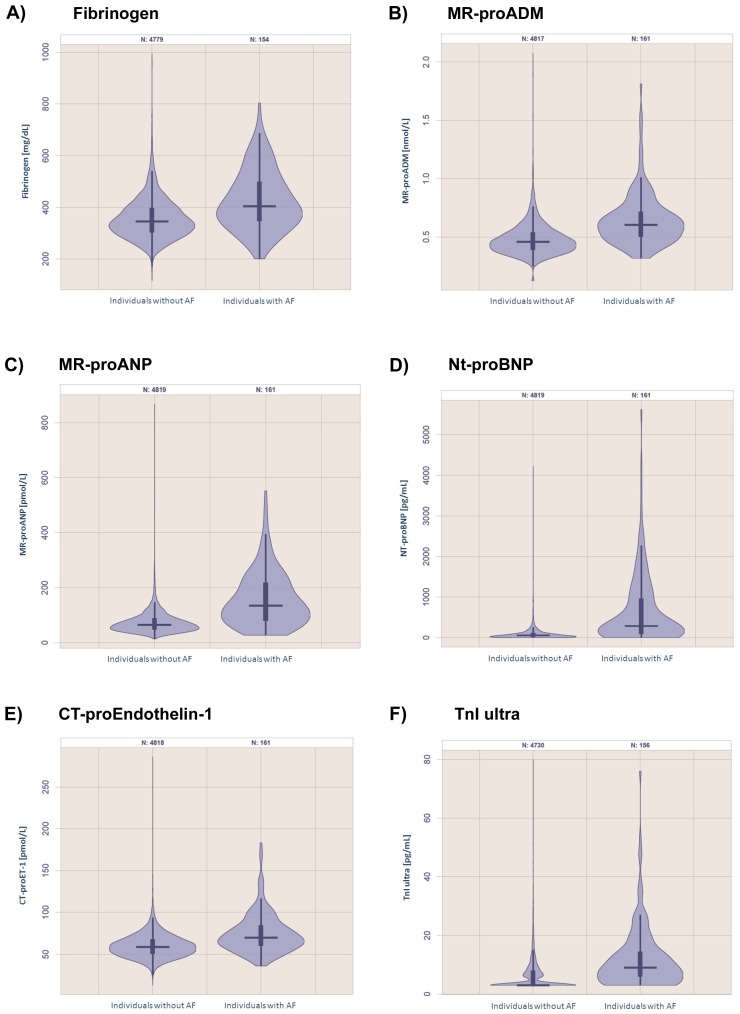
Violin plots of the distribution of circulating biomarkers in the total sample and in individuals with AF for markers that remained statistically significant in relation to AF in multivariable-adjusted models. For presentational reasons some outliers were removed from the plots. For MR-proADM values above 2.5 nmol/L (N = 2), Nt-proBNP values above 8000 pg/mL (N = 3), TnI ultra values above 80 pg/mL (N = 12) were excluded. Abbreviations: AF, atrial fibrillation; CT-proET, CT-pro endothelin-1; MR-proADM, mid-regional pro adrenomedullin; MR-proANP, midregional pro atrial natriuretic peptide; N-terminal pro B-type natriuretic peptide; TnI, troponin.
